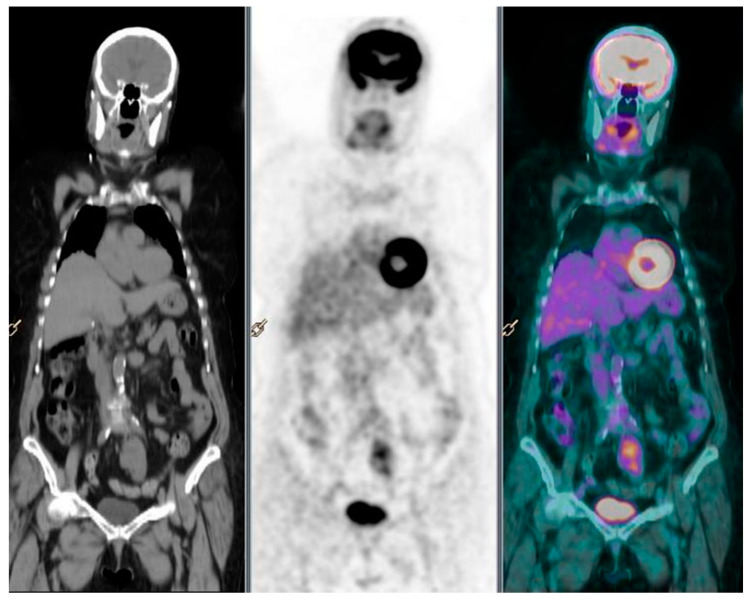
PET/CT scans performed at October 2020: The examination does not show pathologies with a high glucose metabolism.
